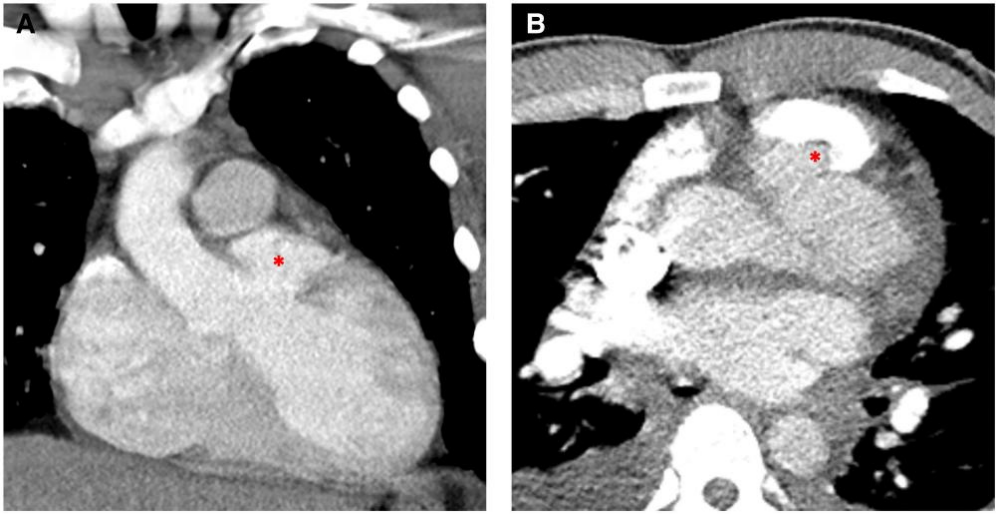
(*A*) Coronal computed tomography reconstruction showing a large pseudoaneurysmal sac (red asterisk) arising from the anterior aspect of the ascending aorta. (*B*) Axial computed tomography confirmed a saccular vascular formation measuring 28 × 33 mm on the left lateral side of the aortic root.
